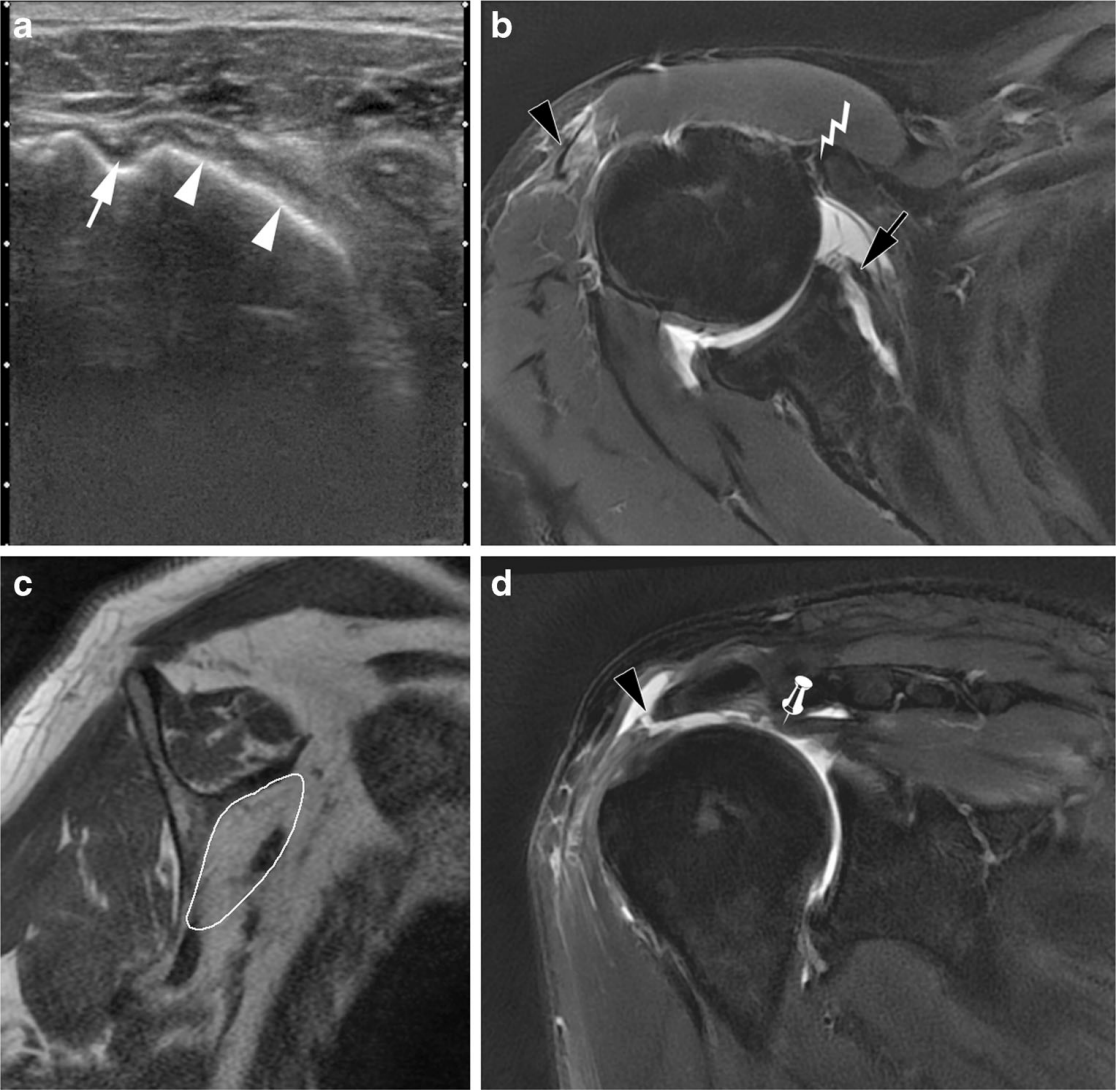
Sixty-two-year old man with a massive right rotator cuff tear involving the SSP, LHBT and SSC tendons. Transverse US image (a): exposure of the lesser tuberosity (white arrow heads) consistent with a complete full thickness tear of the SSC tendon. The intertubercular sulcus (white arrow) is empty because the LHBT is torn. Axial FS DP weighted MRI image (b): proximal retraction of the torn SSC tendon (black arrow). On sagittal T1 weighted MRI images (c) there is severe amyotrophy and fatty degeneration of the SSC muscle (white outline). On coronal FS DP weighted MRI images, there is an intermediate retraction of the torn SSP tendon (pin) (d). As the SSC tendon can no longer fulfil its stabilizing role, there is passive instability and an anterior sub-dislocation of the humeral head coming in contact with the coracoid process (lightning) (b). Also note an associated tear of the acromial head of the deltoid tendon (black arrow heads) (b, d)
